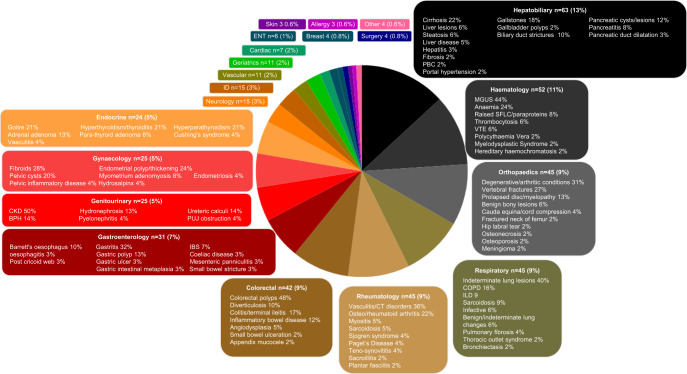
Serious benign conditions (*n* = 480); percentage (%) of patients affected by speciality. Pie chart highlighting the different specialities patients with non-cancer conditions were referred to which are colour coded with percentage volume of overall referalls. The more common specialities have a breakdown of the types of conditions diagnosed. The percentage in the box is by volume of that speciality. BPH benign prostatic hypertrophy, CKD chronic kidney disease, COPD chronic obstructive pulmonary disease, CT connective tissue disorders, ENT ear nose and throat, IBS irritable bowel syndrome, ID infectious diseases, ILD interstitial lung disease, PBC primary biliary cirrhosis, PUJ pelviureteric junction, MGUS monoclonal gammopathy of undetermined significance, SFLC serum free light chain, VTE venous thromboembolic disease.
